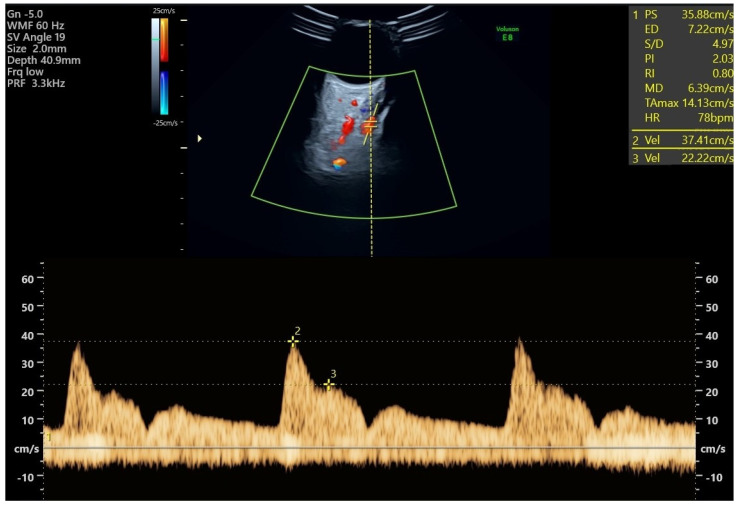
Ophthalmic artery Doppler study: an ultrasound image shows the ophthalmic artery’s color flow. The ocular artery flow velocity waveform, which shows the first and second systolic velocities, is displayed at the bottom and was obtained using pulsed-wave Doppler imaging.
